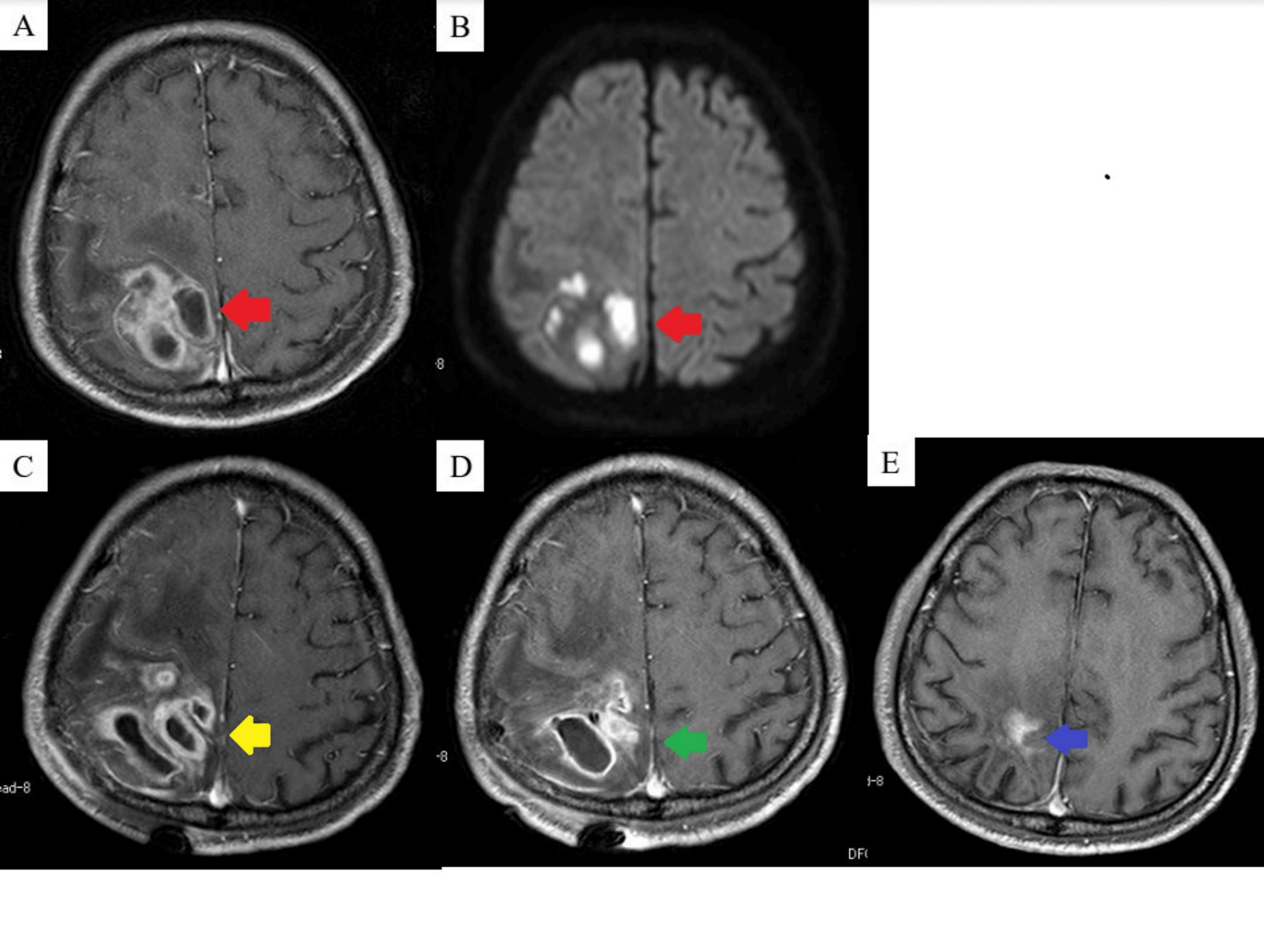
Imaging findings of the representative case (A) MRI T1CE: The red arrow indicates the preoperative abscess. (B) MRI DWI: The red arrow indicates the preoperative abscess. (C) MRI T1CE: The yellow arrow indicates the abscess at first recurrence. (D) MRI T1CE: The green arrow indicates the abscess at the second recurrence. (E) MRI T1CE: Follow-up MRI shows no recurrence (blue arrow). DWI, diffusion-weighted imaging; T1CE, T1-weighted contrast-enhanced MRI
